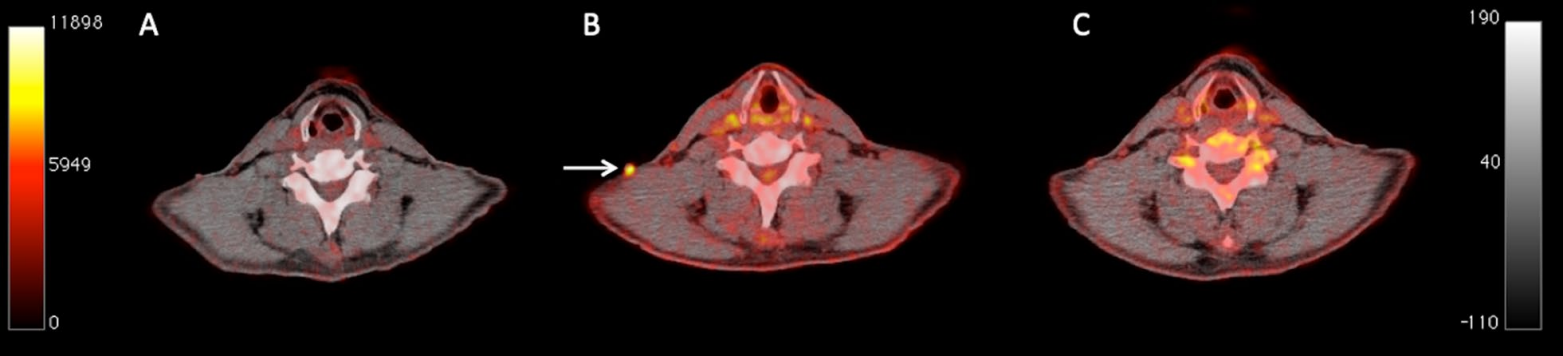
Transaxial PET/CT images at the cervical level of a 55-year-old male melanoma patient undergoing immunotherapy with ipilimumab (a–c). The PET/CT images before immunotherapy showed no pathologic lesions (a). Interim PET/CT performed after two cycles of ipilimumab demonstrated a new, small, ^18^F-FDG-avid subcutaneous lesion in the patient’s neck (white arrow; b), suspicious of metastatic involvement (uPMD, according to EORTC). A third PET/CT obtained soon after administration of four cycles of ipilimumab showed remission of the lesion (c), suggesting pseudoprogression of the cervical finding on interim PET/CT. The application of PERCIMT classified the patient as SMD already at the time of interim PET/CT, thus avoiding misinterpretation. At last follow-up the patient was still alive having reached an OS of 84.4 months
